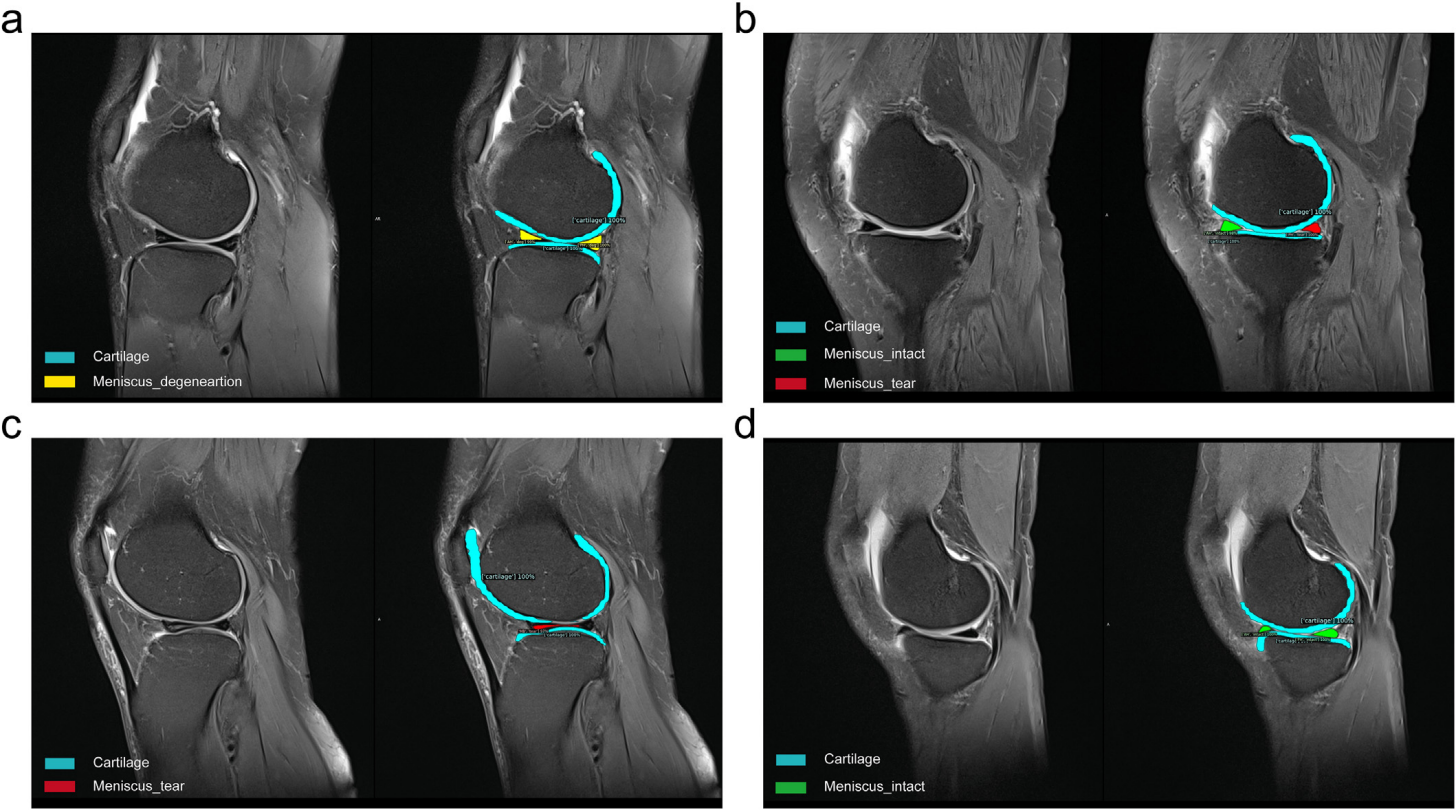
Mask diagnosis results on meniscus MR images. (a) Degenerations at meniscal anterior and posterior horns, (b) Tears at meniscal body, (c) Tears at the posterior horn, (d) Healthy meniscus.
